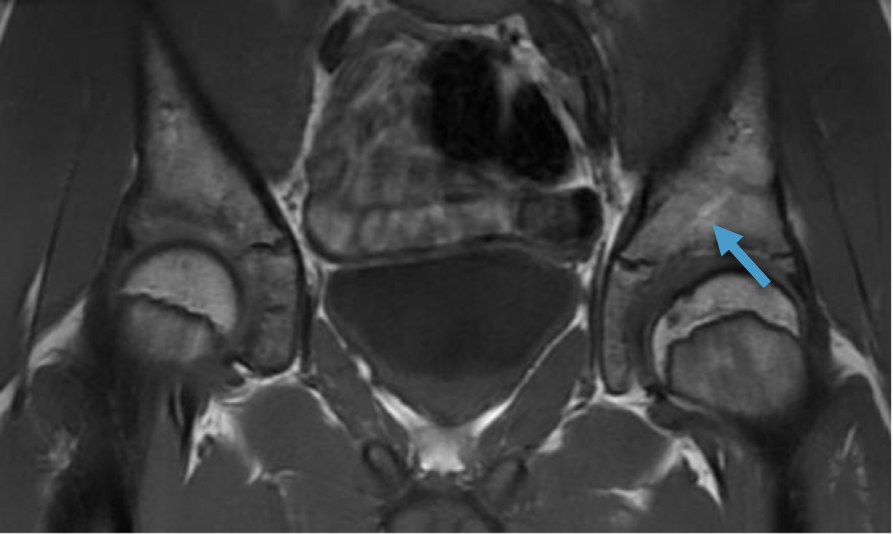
MRI (coronar T1W/3T) on a 12 year-old boy performed 3.6 years postoperatively after a Salter osteotomy due to Perthe’s disease. The screws are mostly replaced with bone but parts of one of the two screws (arrow) still exhibit a mixed signal, i.e. fat signal. This was the only case were the screws were not completely replaced by bone after > 2 years, and this figure shows the section with the most mixed signal
